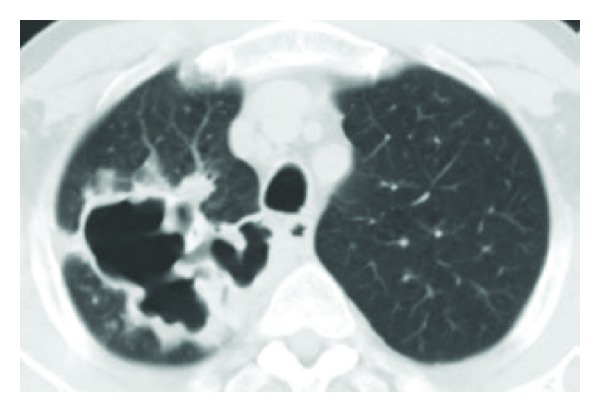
Computed tomography of the chest at presentation.
